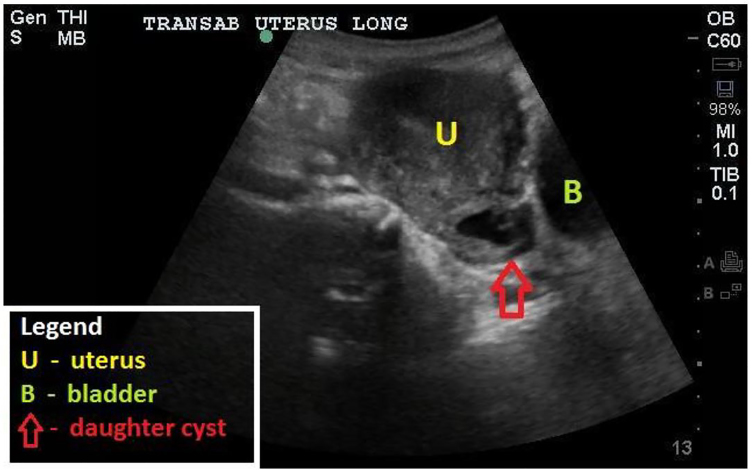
Point-of-care transabdominal ultrasound demonstrating the uterus and daughter cyst in the sagittal plane.
